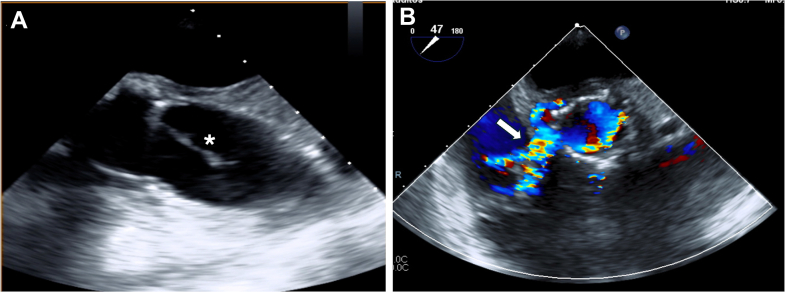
Preoperative Transesophageal Echocardiography (A) Transesophageal echocardiography showing vegetation attached to the aortic prosthesis (asterisk). (B) Color Doppler showing fistulous communication between the noncoronary sinus and the right atrium (arrow).
